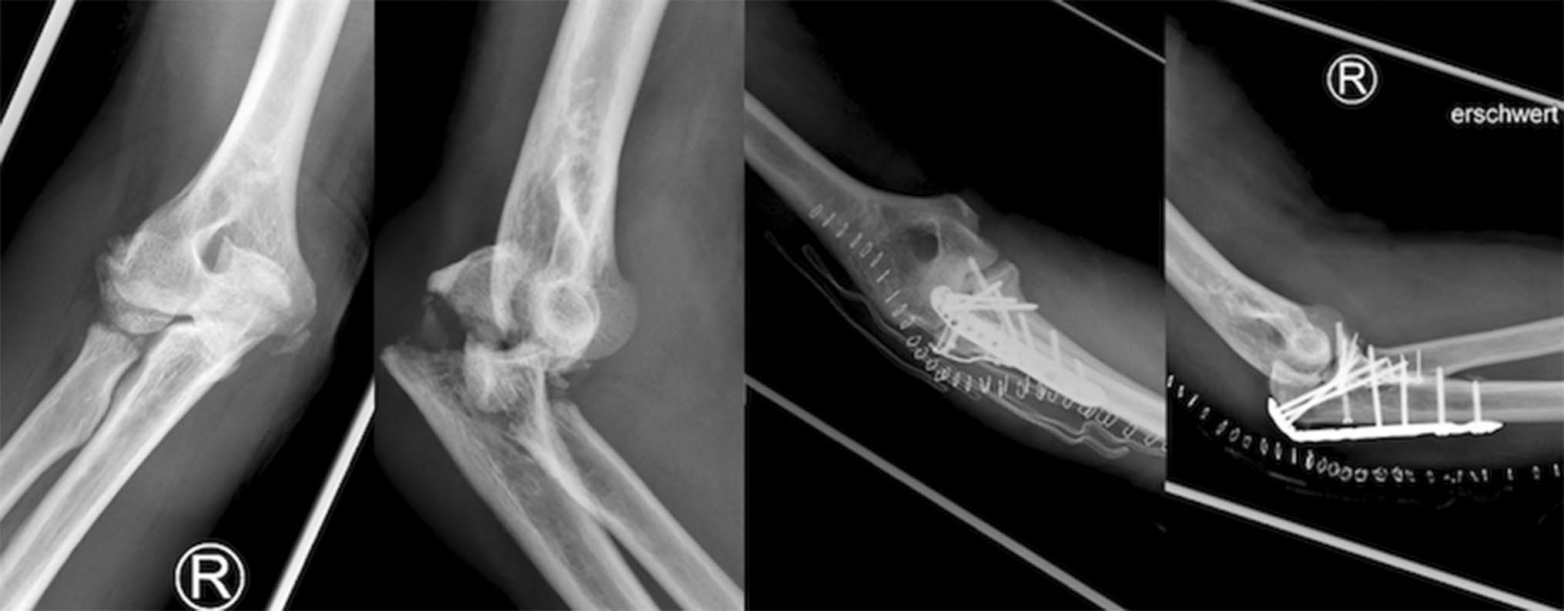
Antero-posterior and lateral radiographs of complex elbow dislocation. a, b Concomitant fractures. c, d Internal fixation with locking plates and screws
